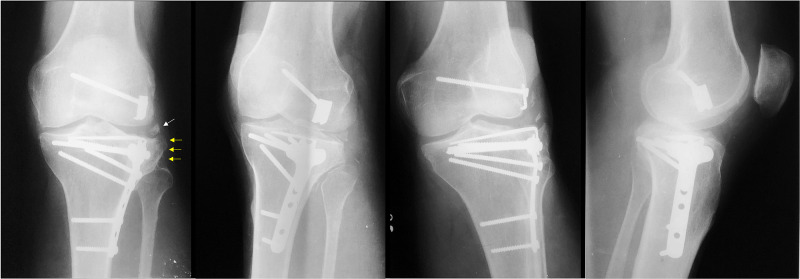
GM, 35-year-old, male patient, fall from a motorcycle Postoperative X-rays of the left knee at 24 months – there were slight peripheral subsidence and widening of the lateral tibial plateau (yellow arrows). Also, an isolated osseous fragment can be noted, which we attributed to heterotopic ossification on the lateral collateral ligament (white arrow).
